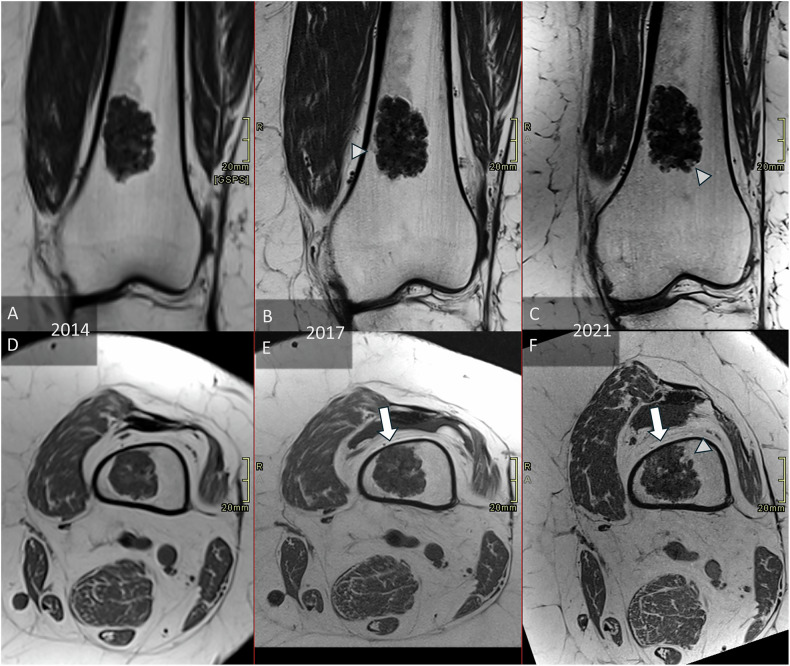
TSE T1-weighted coronal and axial images of LG-CCL (A, D) with new scalloping (E, F arrow) and signs of increasing fat entrapment over time and fat replacement (B, C and F, arrowhead). Cranio-caudal length has been measured on different slices focusing on maximum lesion size and is therefore not shown here. LG-CCL, Low-grade central cartilaginous lesion
